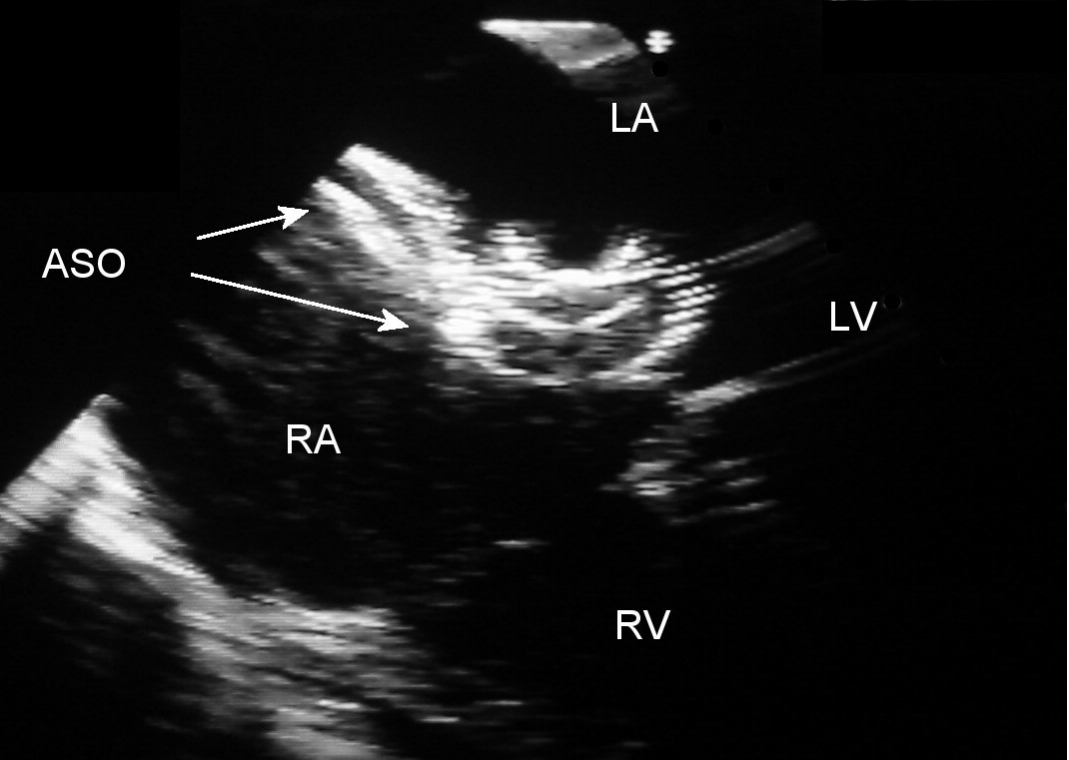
Transesophageal echocardiography four-chamber image following deployment of the two Amplatzer septal occluders (ASO). LA – left atrium, LV – left ventricle, RA – right atrium, RV – right ventricle.
